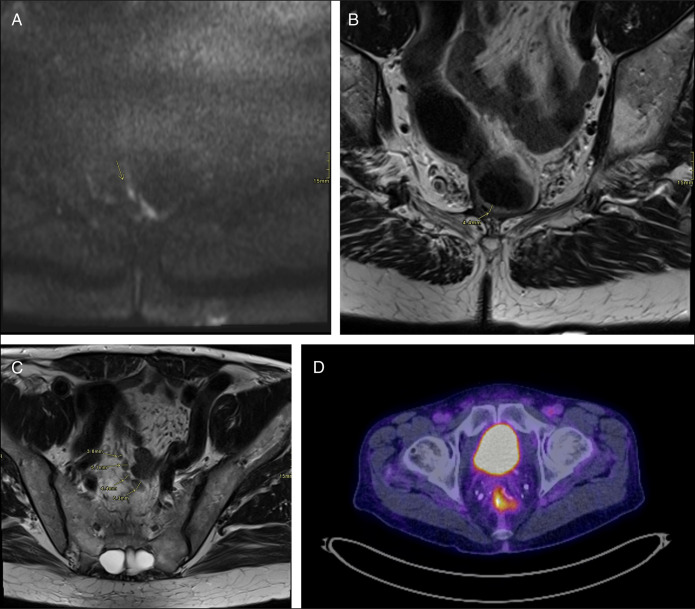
(A, B) Crescent of restricted diffusion along the area of PSMA uptake in the right lateral J-pouch (arrow) and is associated with an area of thickening on the axial T2 image. (C) Prominent mesorecctal nodes (arrows). (D) Relevant PSMA positron emission tomography image. Surgical change of J-pouch with nonspecific uptake along the right and inferior J-pouch wall. PSMA, prostate-specific membrane antigen.
